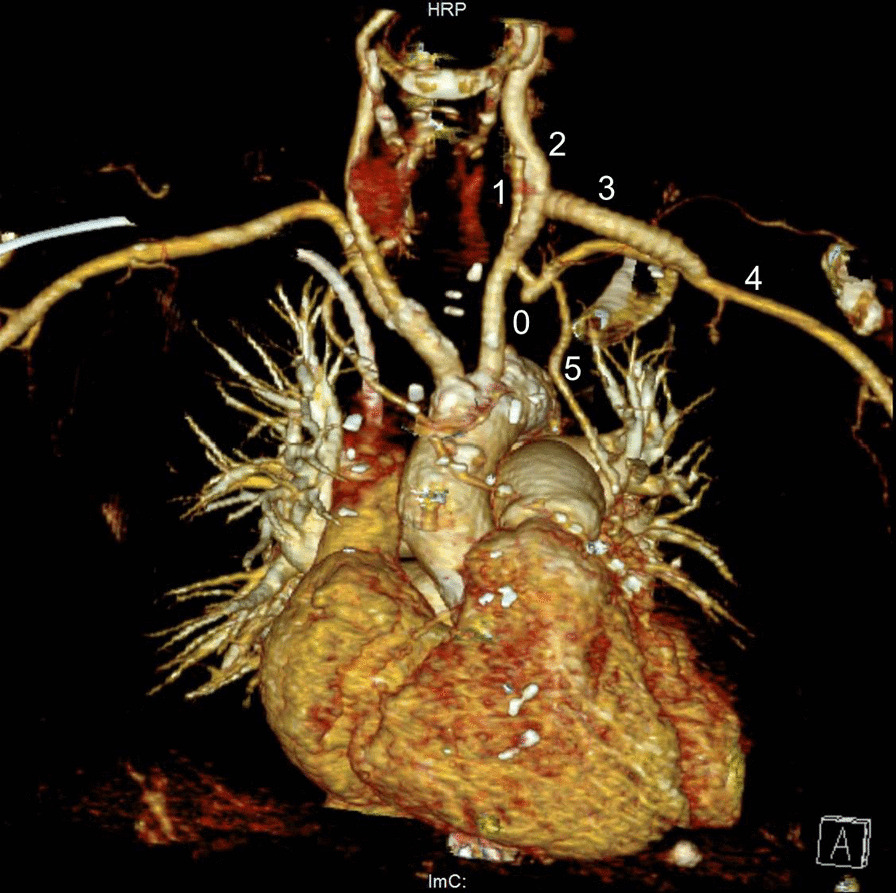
3D image reconstruction of the postoperative CT-angiography control, showing the vascular reconstruction with the proximal left subclavian artery occlusion (0), the left vertebral artery (1), the left common carotid artery (2), the carotid-subclavian bypass (3), the left subclavian artery (4), and the patent coronary artery bypass graft, performed using the left internal mammary artery (5)
